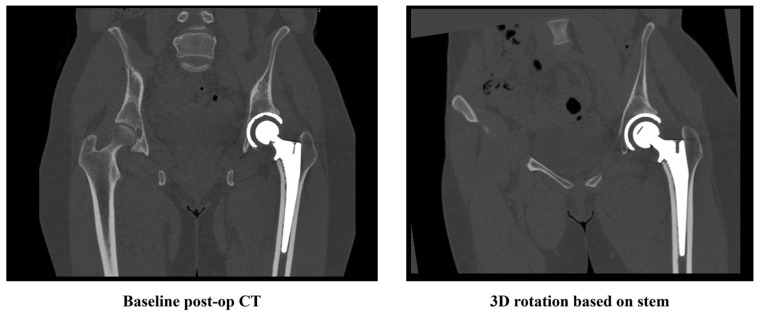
Screenshot of a CT slice image before and after applying 3D rotation based on the stem coordinate.
